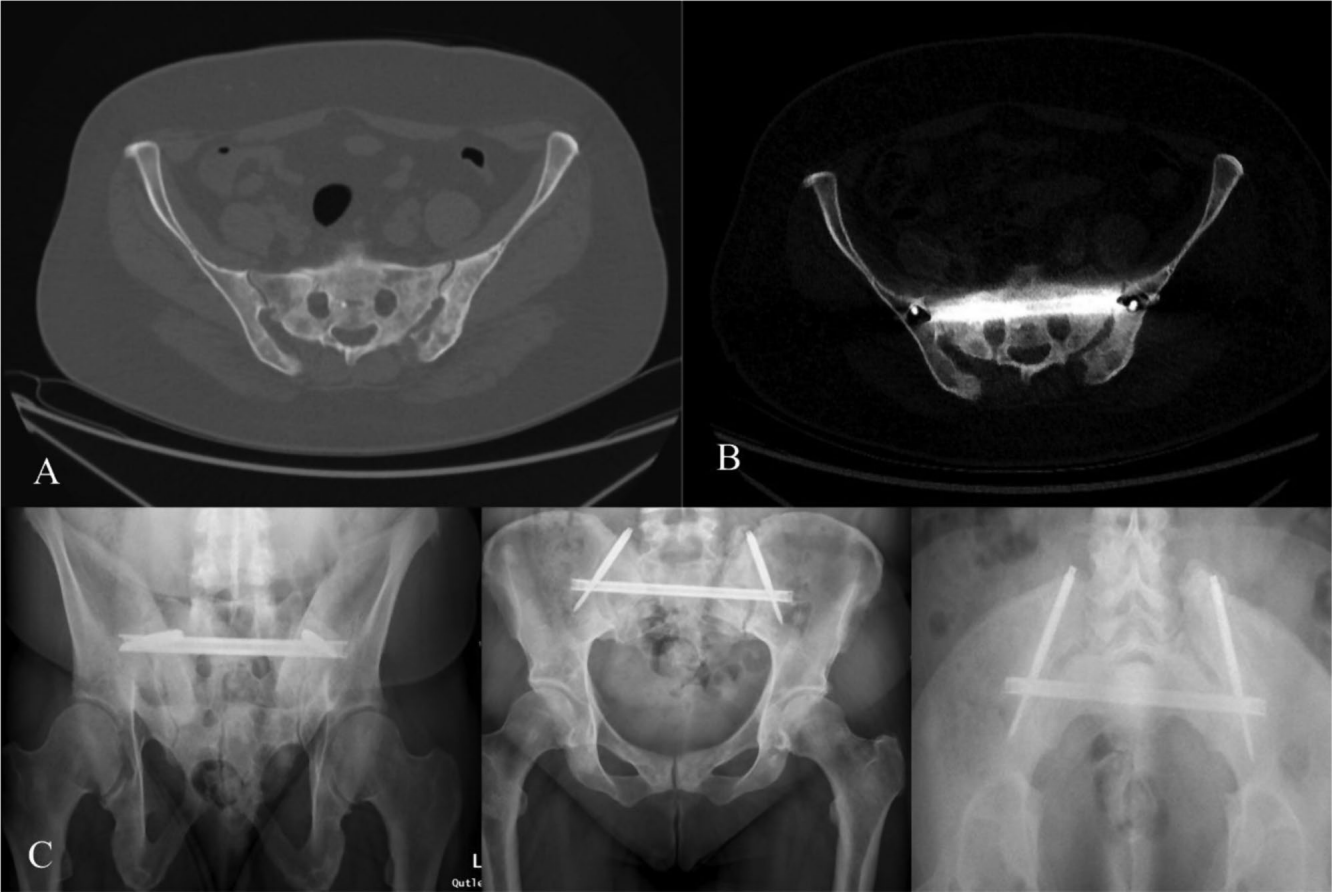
Patient 2 A- preoperative CT scan showing sacral fracture, B- postoperative CT scan, C- X-rays demonstrating no implant failure 12 months postoperative
